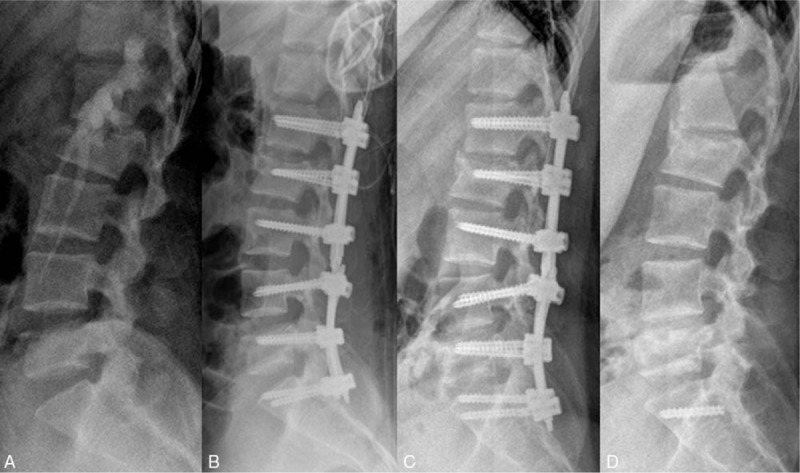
Change of Cobb angle in serial lateral radiographs. (A) Before surgery, the Cobb angle of L2 was 3.8° and that of L5 was −32.8° (B) After immediate surgery, the angle was reduced to −7.5° and −36.1° (C) At 12 months after surgery, the angle changed to 6.1° and −15.6° (D) At 24 months after surgery, the angle increased to 13.3° and −15.5°.
